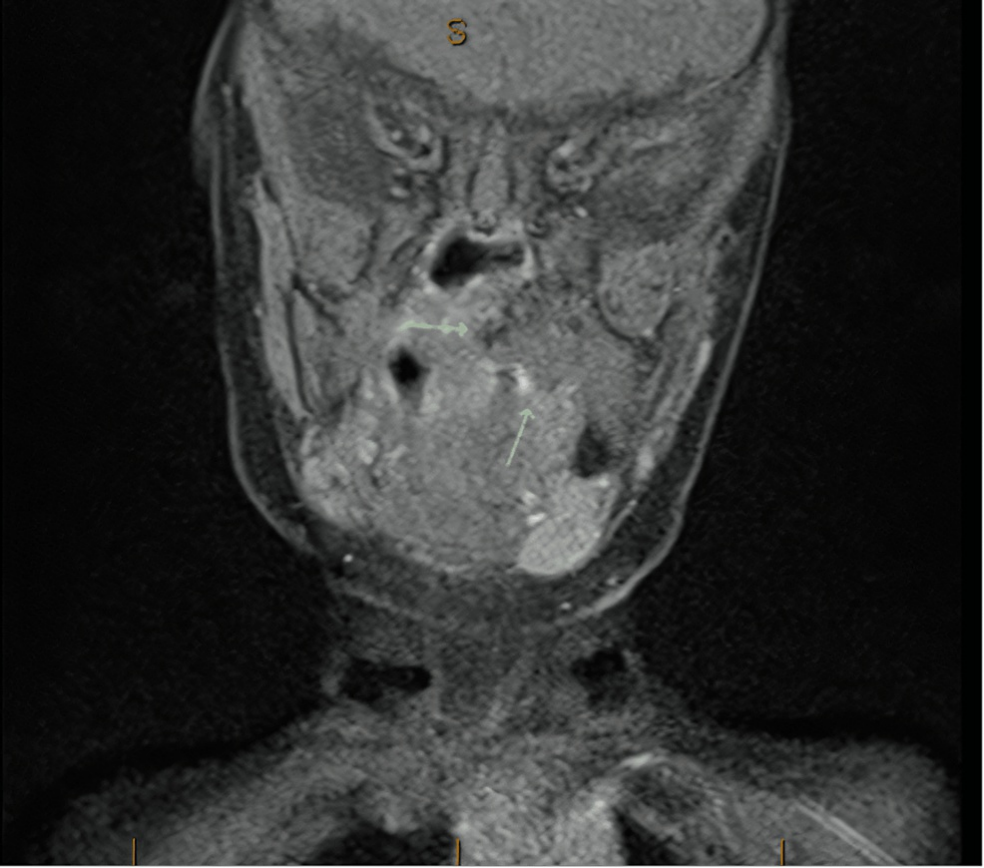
MRI brain T1-weighted post contrast
